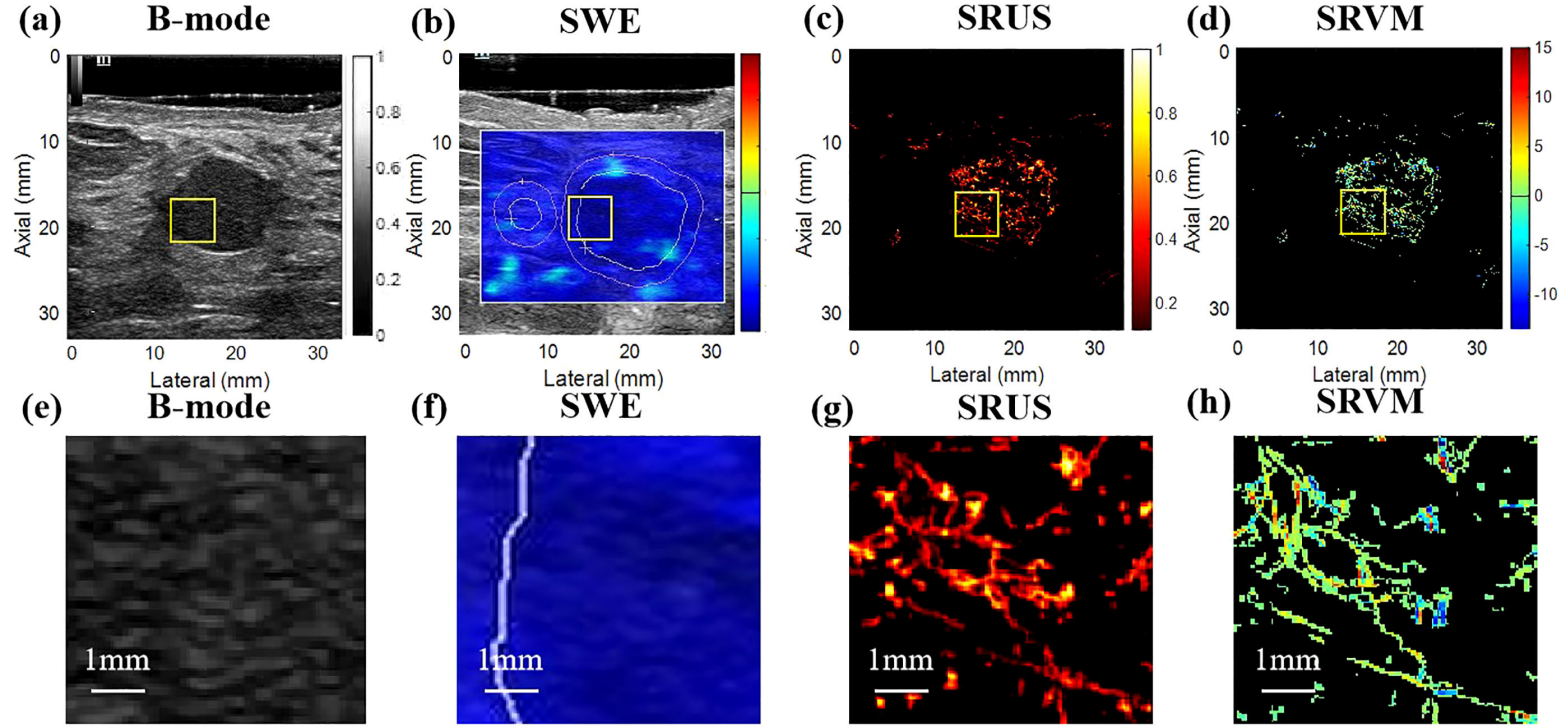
Ultrasound images of benign breast masses. (A) B-mode; (B) shear-wave elastography; (C) super-resolution ultrasound imaging; (D) super-resolution velocity map. (E–H) were the details of the breast mass between (A) B-mode, (B) shear-wave elastography, (C) super-resolution ultrasound imaging, and (D) super-resolution velocity map, as shown in the yellow box in the figure.
